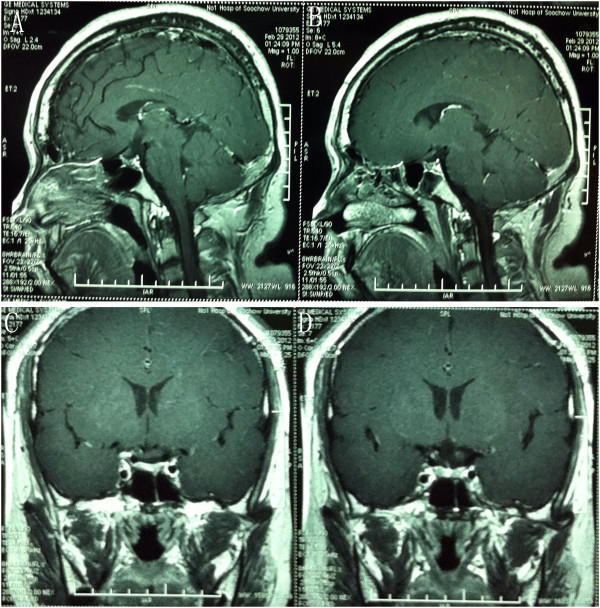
Case number 13: Axial (A-B) and coronal (C-D) view of contrasted magnetic resonance imaging showing total removal of craniopharyngioma.
